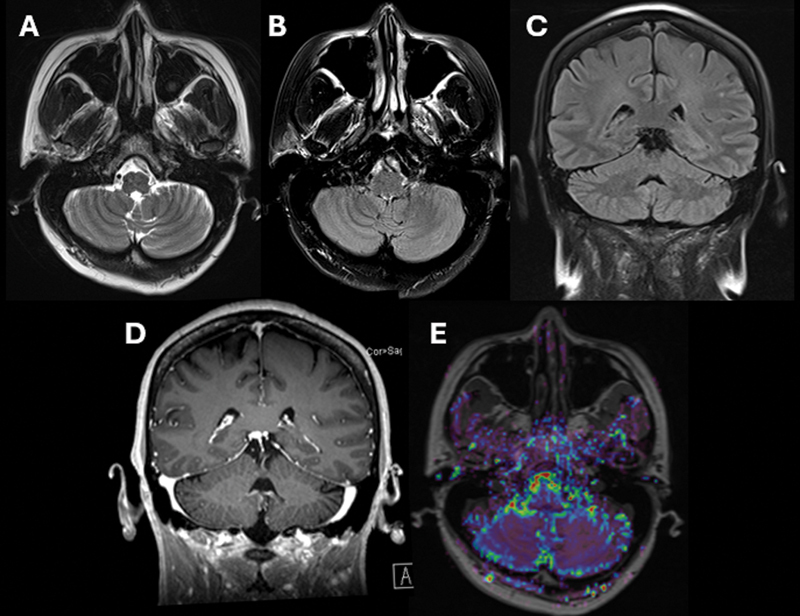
Follow-up brain MRI scan performed a few months later. (
